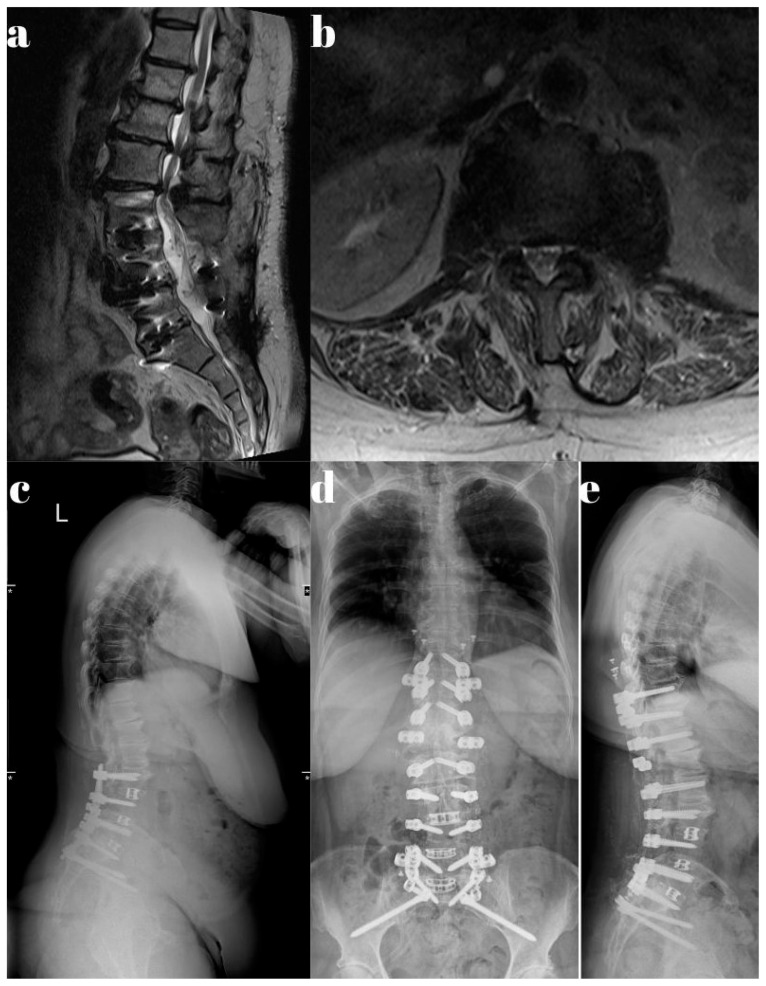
A patient who previously underwent L3-iliac rigid stabilization developed adjacent segment disease and presented with severe left leg and back pain. MRI revealed multilevel stenosis from T11–L3. (a) Sagittal T2-weighted MRI showing multilevel spinal stenosis. (b) Axial T2-weighted MRI demonstrating foraminal stenosis. (c) Preoperative full-spine lateral X-ray showing L3-iliac rigid stabilization with three-level TLIF instrumentation. (d) Postoperative full-spine coronal X-ray displaying dynamic stabilization. (e) Postoperative full-spine lateral X-ray demonstrating dynamic stabilization.
